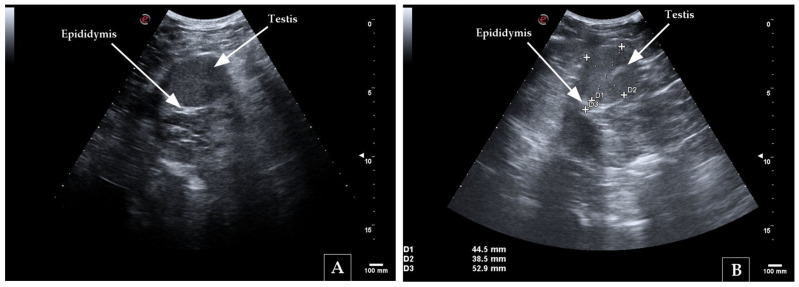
Ultrasonographic images of intra-abdominal testes in two bilateral cryptorchid rams. (A) Retained testis visualized within the abdominal cavity; (B) Testis with linear measurements (D1–D3) and identification of the epididymis. The scale on the right indicates 100 mm.
